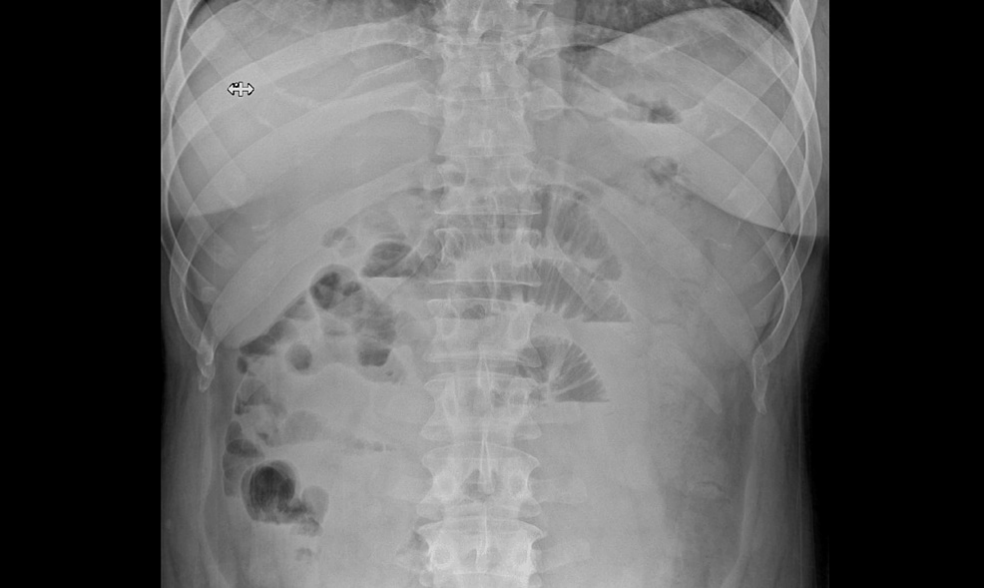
Abdominal X-ray showing multiple air-fluid levels and distended small bowel loops
